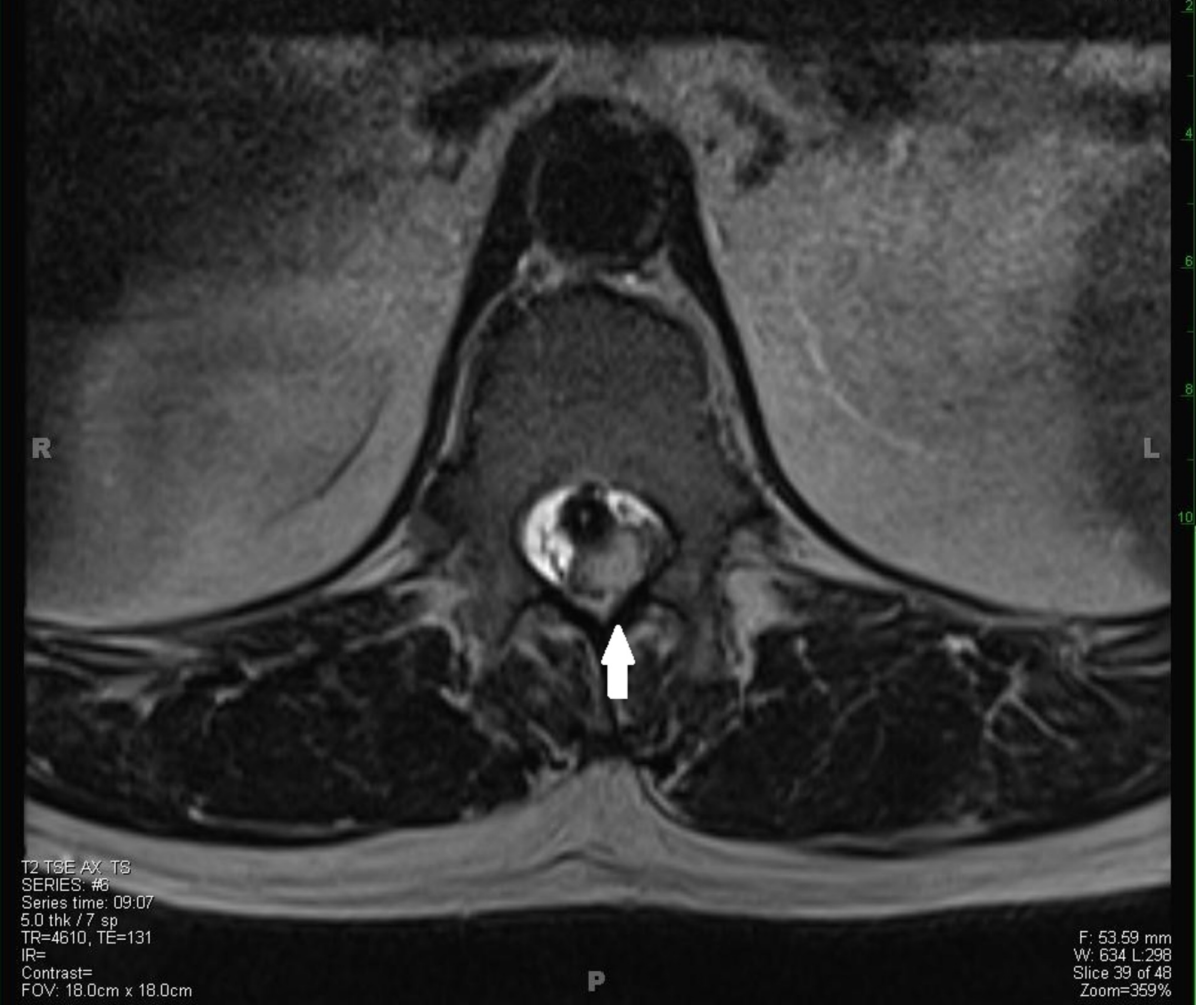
T2-weighted axial MRI showing a 2x1x1 cm intradural lesion at T11-T12 with impingement and compression of thoracic cord and associated edema producing a mass effect. T, thoracic.
